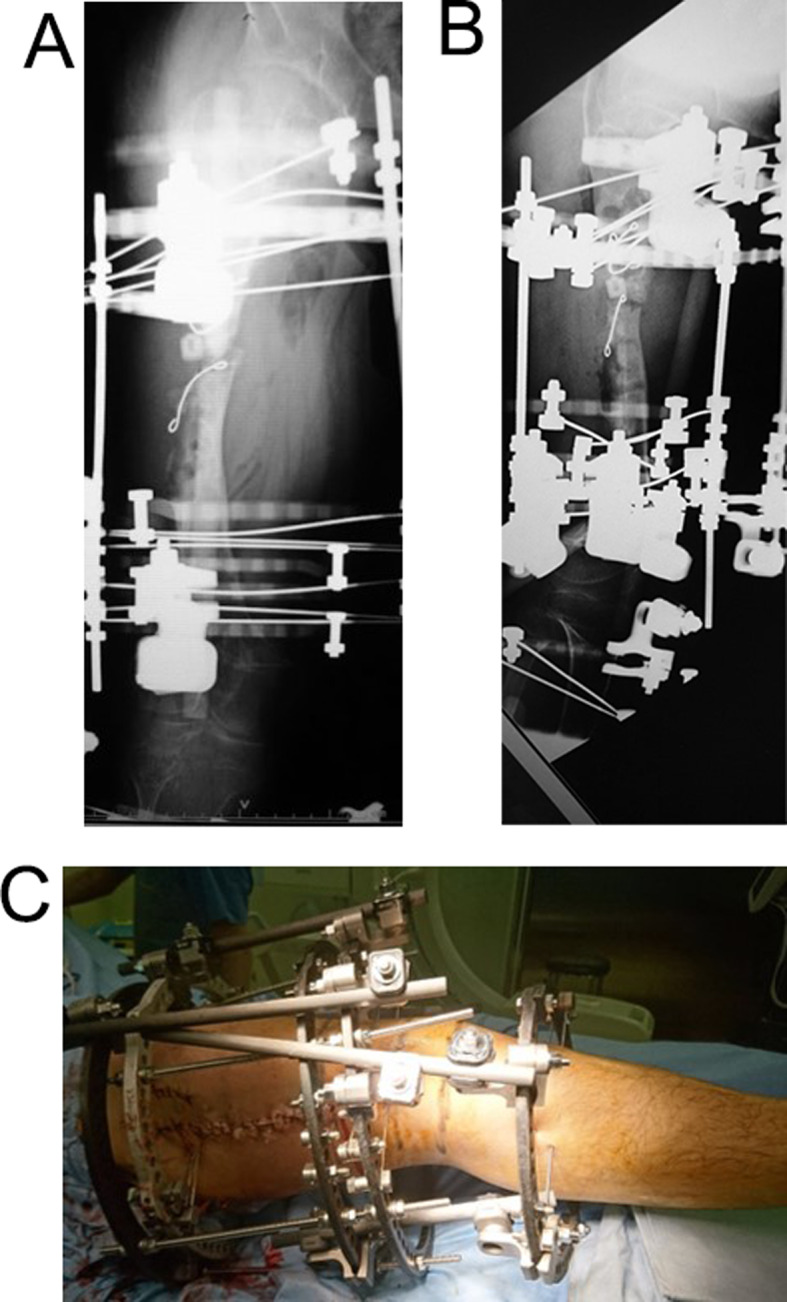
A, B: Immediate postoperative X-ray at initial surgery. C: A knee joint-spanning circular external fixator was used. The tibial ring was removed 2 weeks after surgery, and range-of-motion exercises were started
